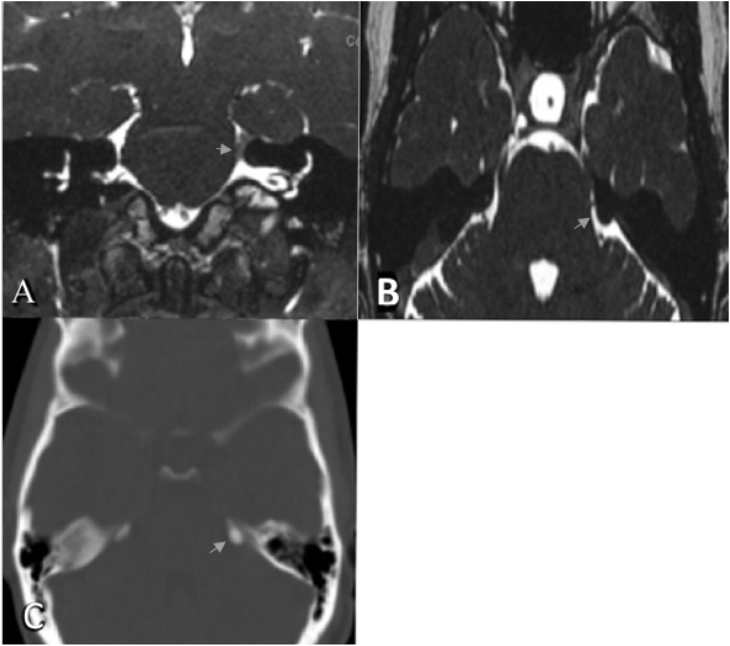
A) and B): Preoperative MRI brain axial and coronal T2 CISS showing left EST (arrows) in close proximity to trigeminal nerve REZ. C) CT scan brain axial cut bone window showing left prominent suprameatal tubercle (arrow).
